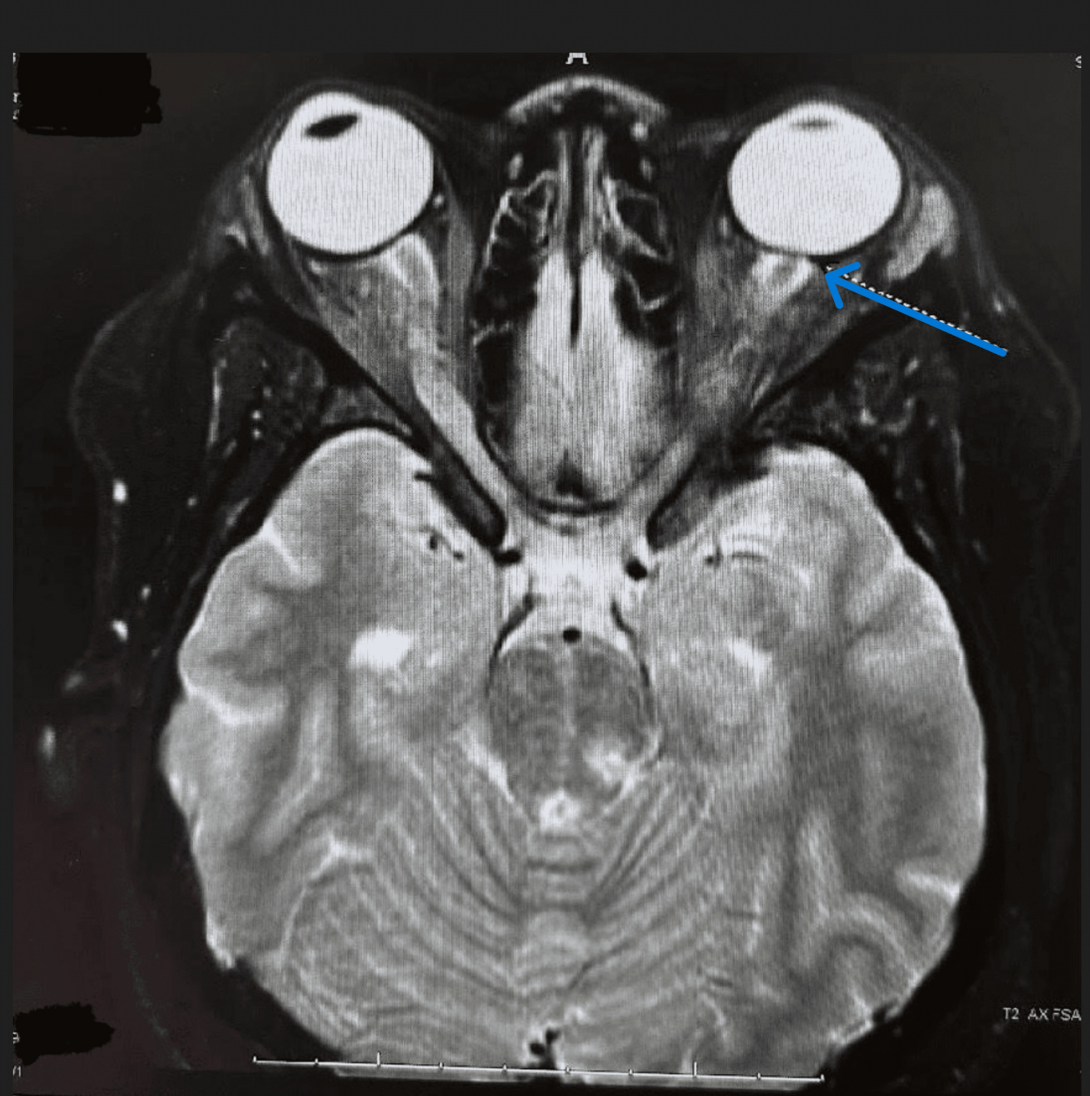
MRI of the brain: tortuous right optic nerve Blue arrow highlighting increased signal of the left optic nerve MRI: magnetic resonance imaging
